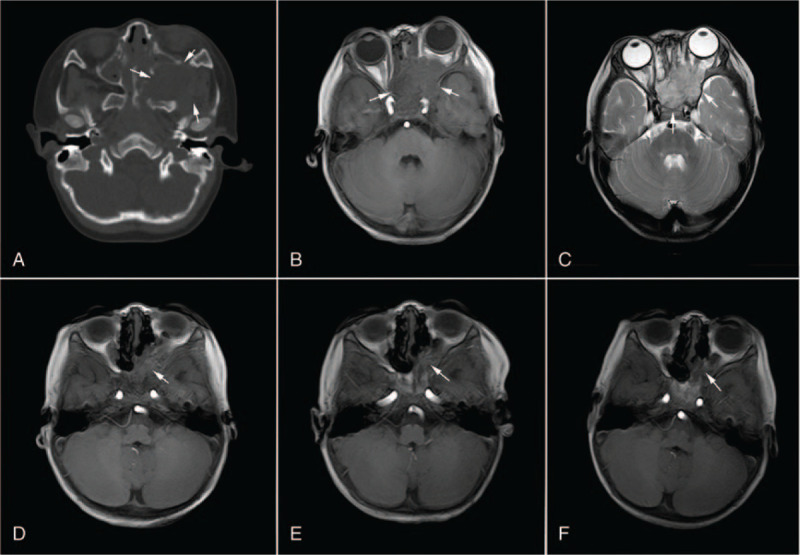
Dynamic changes of yolk sac tumor of pterygopalatine fossa on computerized tomography and magnetic resonance imaging before and after surgery in combination with chemotherapy. A: Tumor mass is accompanied by peripheral bone destruction around the left pterygopalatine fossa before surgery (white arrow); B and C: Yolk sac tumor of the left pterygopalatine fossa with obstructive changes in the sphenoid, ethmoid, left maxillary sinuses, left nasal cavity, and left orbit (white arrow), and the bilateral optic nerve was invaded; D: Residual tumor mass after massive resection and 3 cycles of chemotherapy; E, Tumor mass at the end of 6 cycles of chemotherapy; F, Tumor mass 18 months after index admission.
